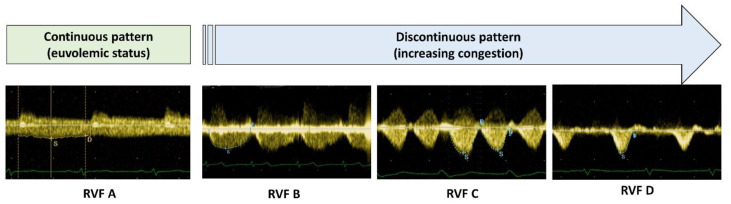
Assessment of renal venous flow (RVF) using Doppler ultrasound. The transition from a continuous RVF (pattern A) to discontinuous (pattern B to D) is an expression of worsening renal congestion.
